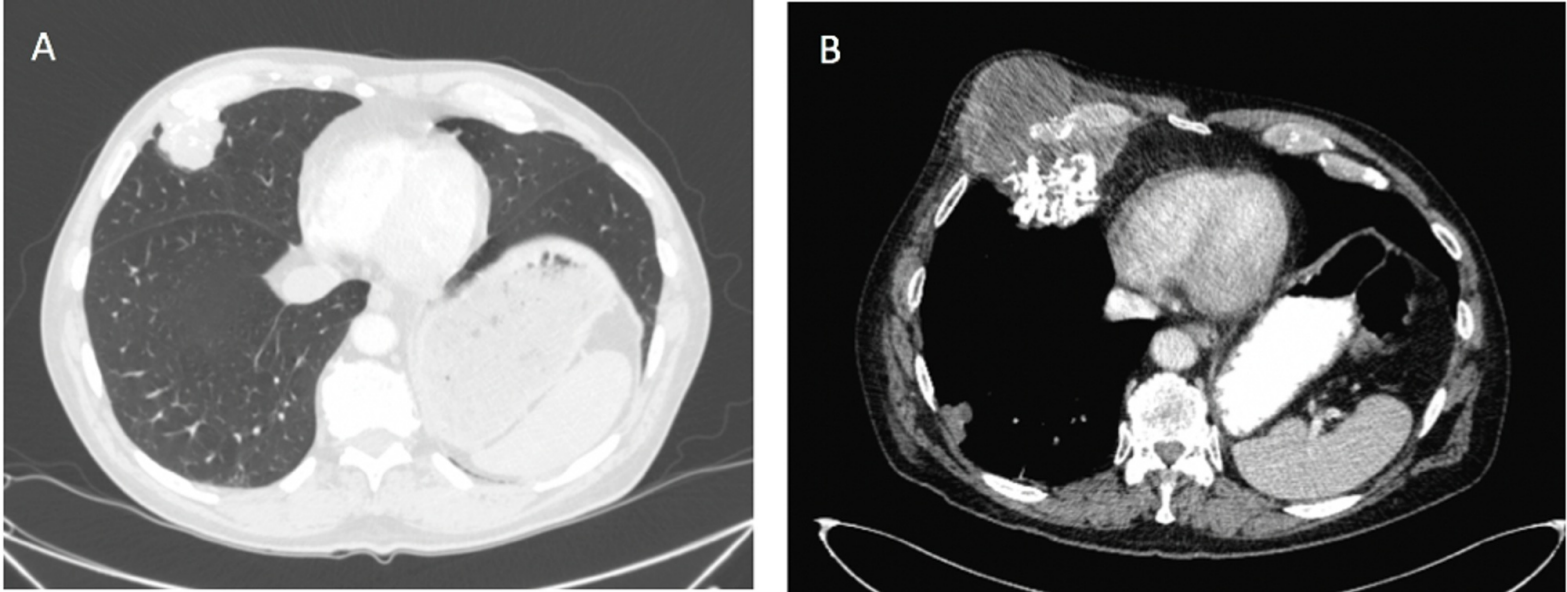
The patient’s right middle lobe mass demonstrated on CT in 2012 (A) and in June 2018 (B).
